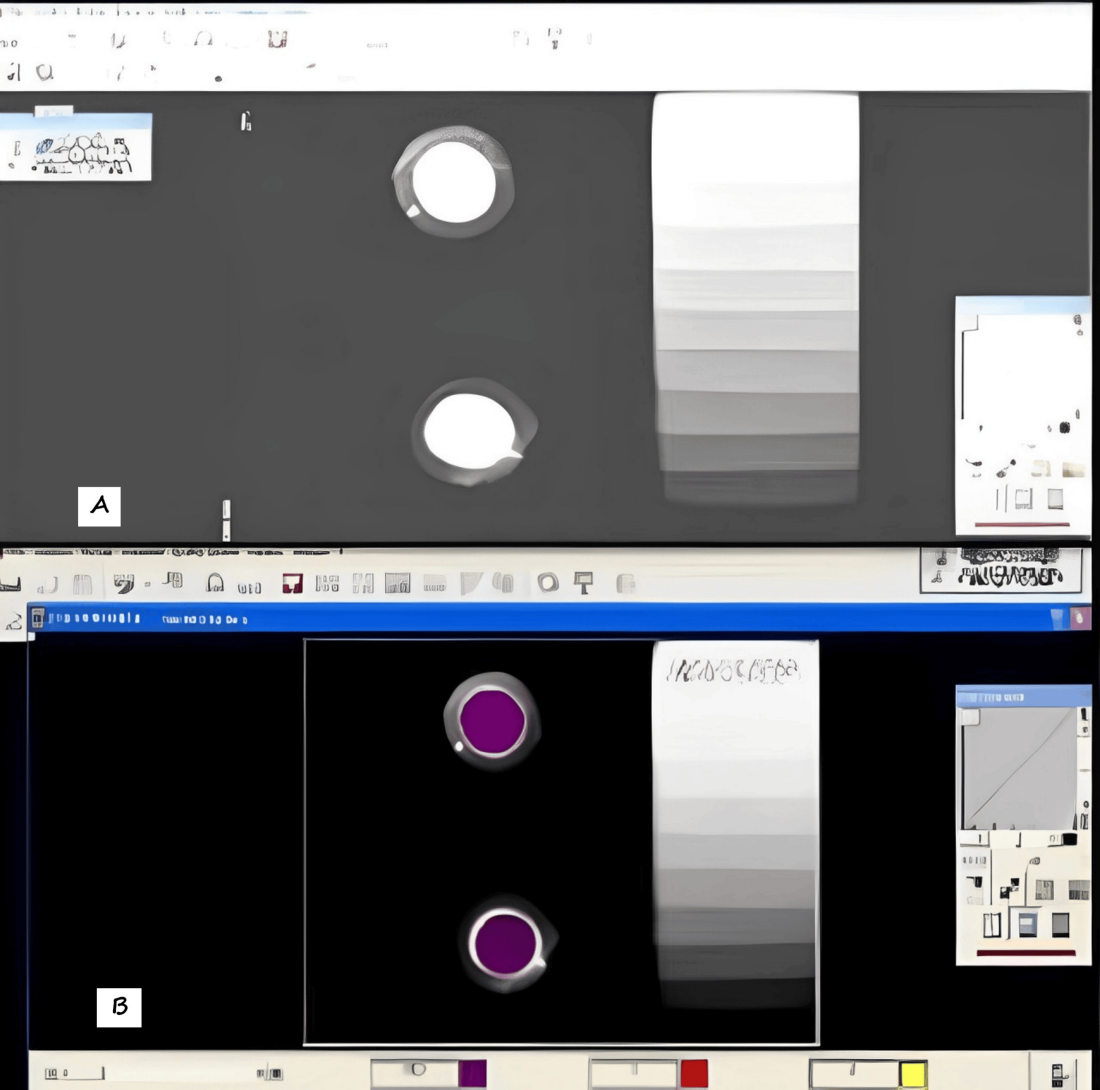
Materials were radiographed separately alongside an aluminium step wedge A: step wedge with the black and white contrast image of the material used B: step wedge with the color contrast image of the material used
